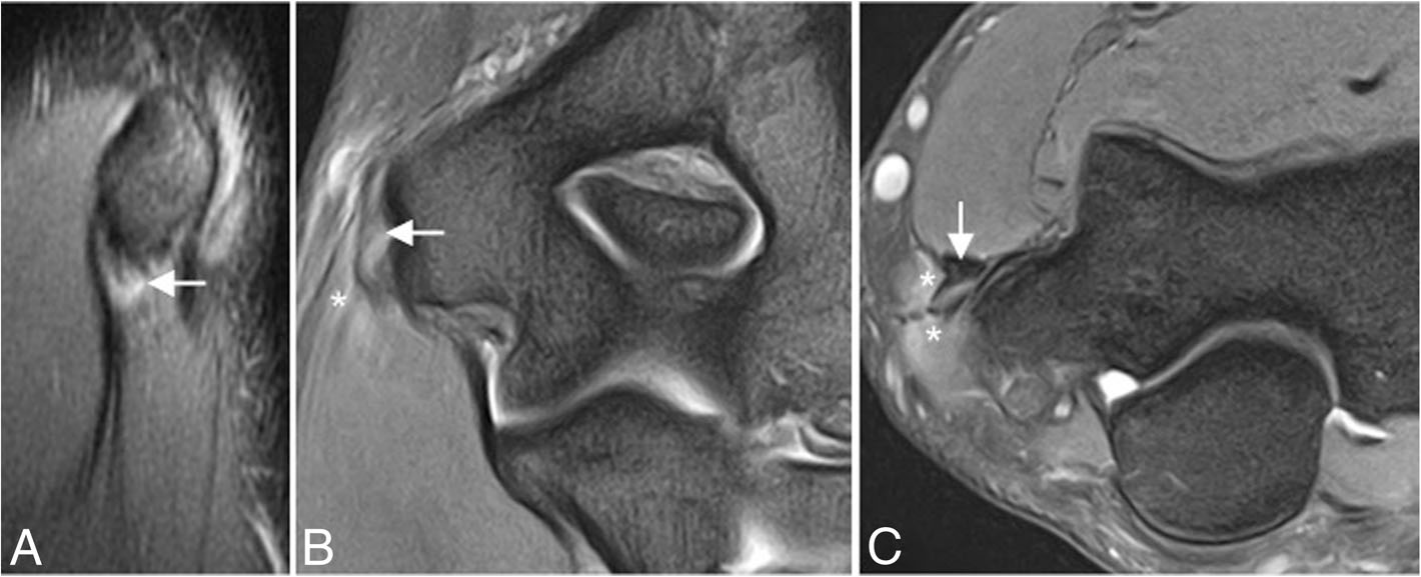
A 45-year-old man with medial elbow pain. Sagittal PD-weighted MRI (a), coronal FS PD-weighted MRI (b), and axial FS PD-weighted MRI (c) showing a common flexor tendinosis with intermediate signal within a diffusely thickened flexor tendon origin (white arrows). Note flexor digitorum superficialis and palmaris longus muscle edema (white asterisks)
